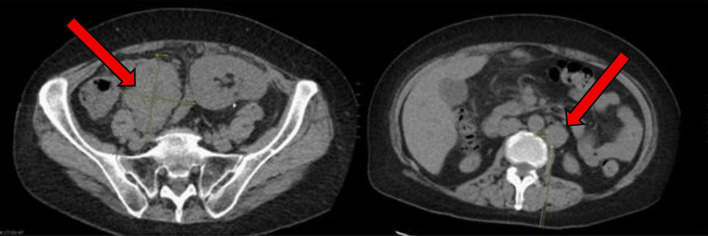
At time of initial presentation, CT abdomen and pelvis showing increase in size of the abdominal and pelvic lymph nodes (arrows) suspicious for post-transplant lymphoproliferative disorder. CT: computed tomography.
